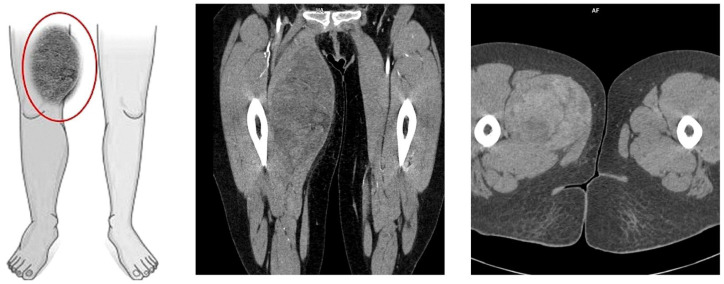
Picture of the tumor’s location and axial/coronal computed tomography imaging of a massive right thigh tumor—red circle (SOMATOM Edge Plus 128-layer CT scanner).
